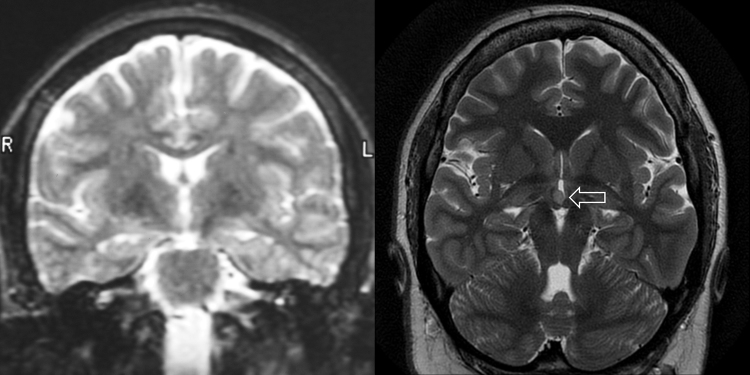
Complex motor seizures due to a hypothalamic hamartoma. Patient with complex motor seizures from the age of 2 years. Imaging at 1.5 T at the age of 22 years in 2001 was reported as normal (A) with the hypothalamic hamartoma only being identified on 3 T imaging in 2011 (B). Image A is coronal T2-weighted images at the level of mammillary bodies and temporal stems and image B is a coronal oblique PROPELLER (periodically rotated overlapping parallel lines with enhanced reconstruction) sequence. Gelastic seizures were identified in hindsight and the patient has undergone gamma knife surgery.
